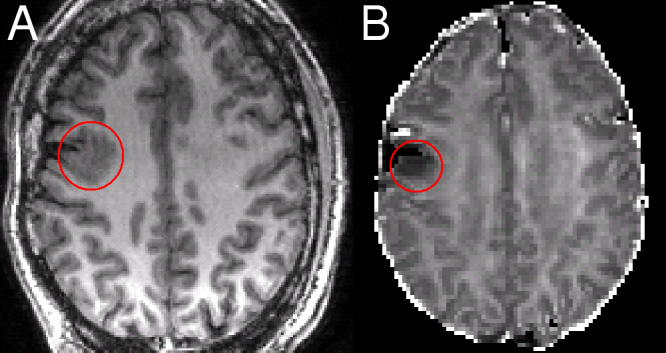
Residual FCD following a right middle frontal gyrus resection. Patient 1: the residual FCD on the T1-weighted image (A) corresponds with an area of reduced ICVF (B).
